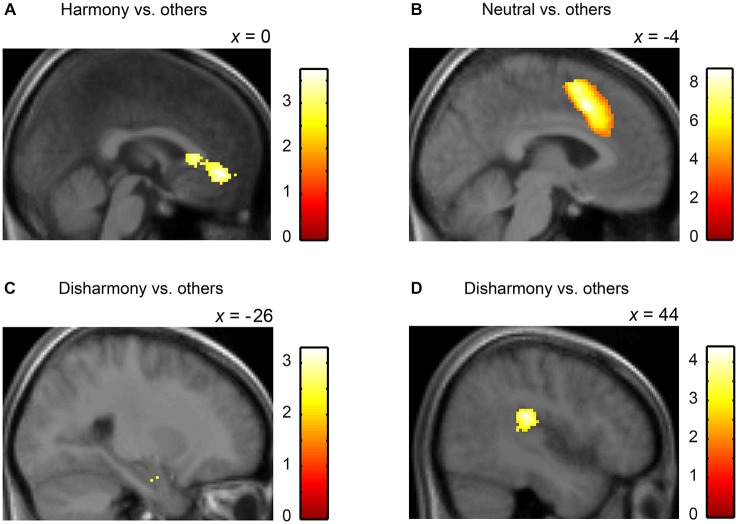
Statistical parametric maps rendered on a mean T1-weighted image (*N* = 18). Harmony vs. others: (A) left medial orbitofrontal cortex and anterior cingulate cortex (Talairach coordinates: 0, 39, −4). Neutral vs. others: (B) left anterior cingulate cortex (−4, 14, 40). Disharmony vs. others: (C) left amygdala (−26, −7, −18) and (D) right posterior insula (44, −28, 22).
